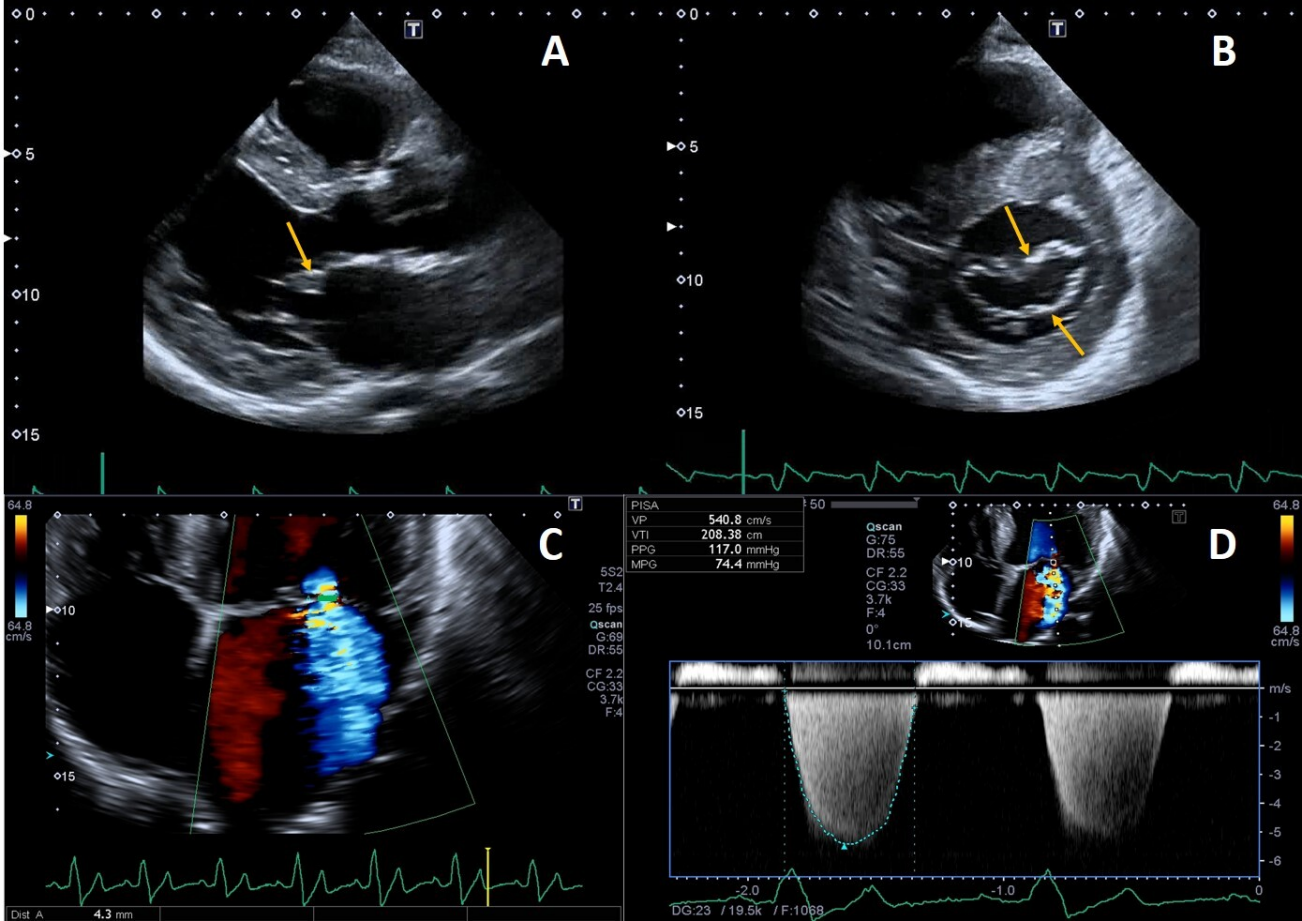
Example of valvular heart involvement in Fabry Cardiomyopathy. (A, B) Parasternal long-axis and short-axis views showing thickened mitral valve
